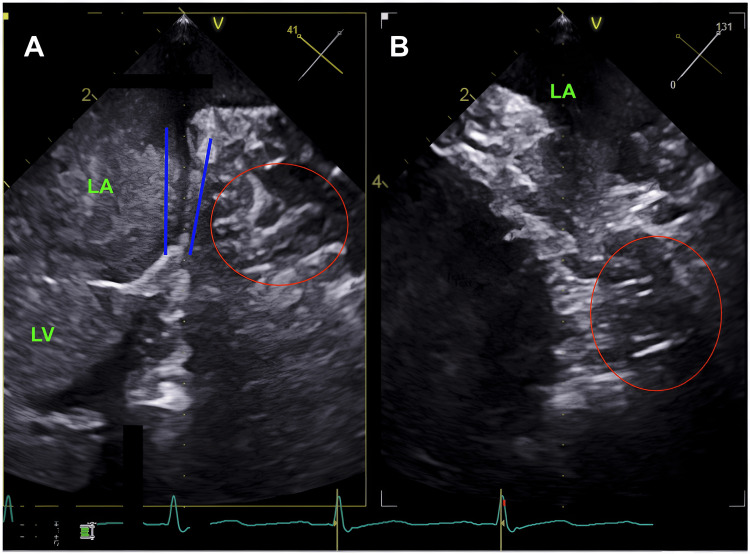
Transesophageal echocardiogram (TEE) of left atrium at 41° and 131° oblique angles LA = Left atrium, LV = Left ventricle, red circle = Congenital absence of left atrial appendage (LAA). (A) 41° oblique image shows contrast (gray behind the "LA" and "LV") filling the left atrium and left ventricle. The blue lines outline the sharp demarcation of where the LAA should connect with the LA, and the area next to the LA shows no filling within the theoretical space of the LAA, supportive of congenital absence. The red circle outlines this hypoechoic chamber, which is anomalous in relation to where the LAA should be. (B) 131° oblique image shows another angle confirming a hypoechoic structure where the left atrial appendage should be, which is highlighted by the red circle.
